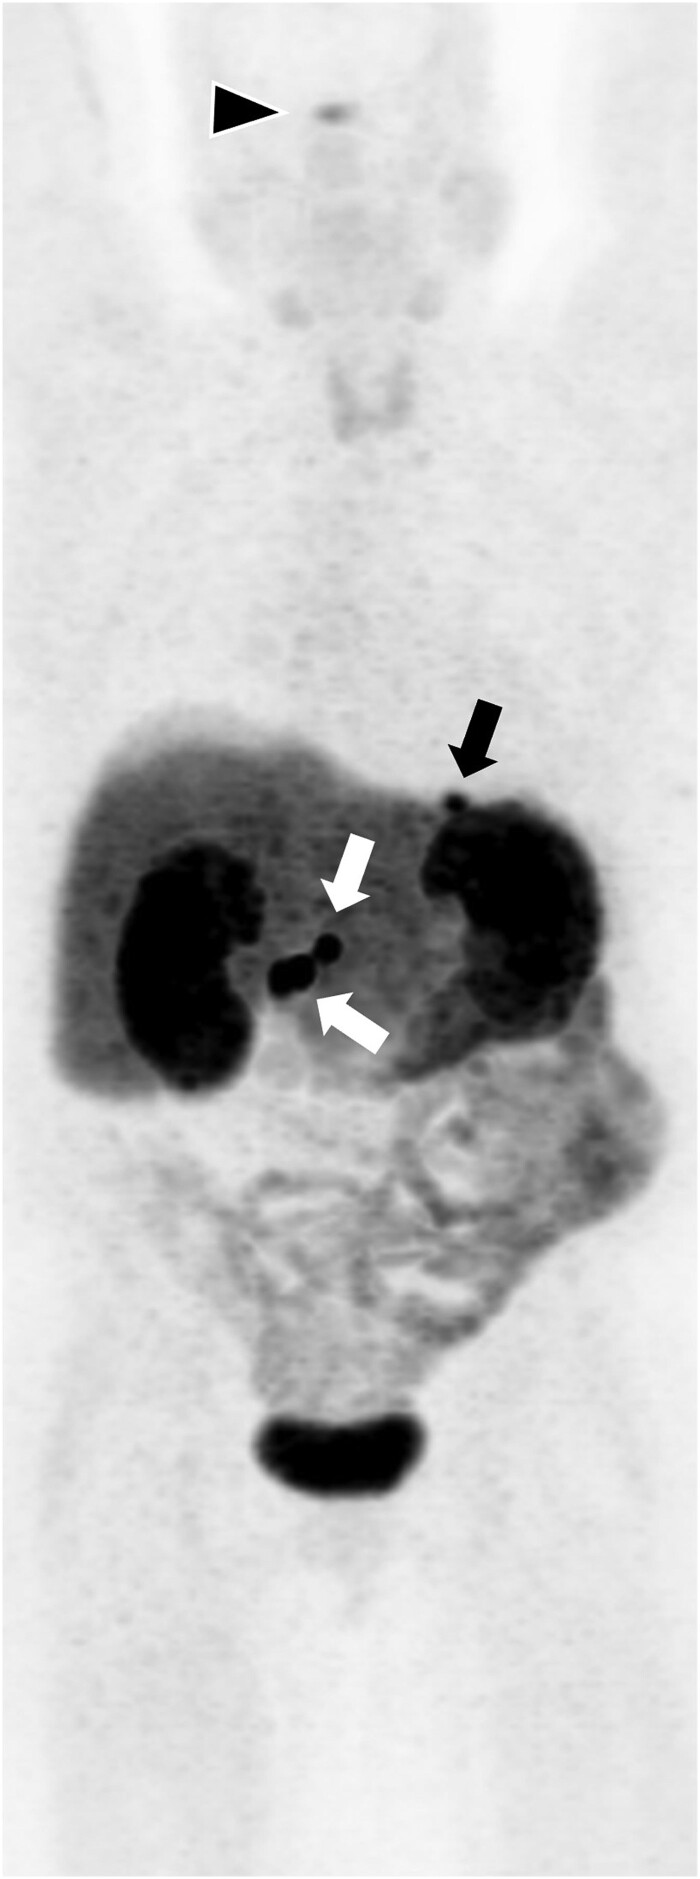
Maximal intensity projection image from the initial Ga-68 DOTA-TATE PET/CT obtained after pancreatic tumor resection demonstrates several somatostatin receptor–positive peripancreatic and porta hepatis lymph nodes (SUV max up to 27.1), measuring up to 1.3 cm in maximal dimension (white arrows). Increased tracer uptake is also seen in a small hepatogastric ligament lymph node (black arrow). Physiologic tracer uptake is seen in the pituitary gland (black arrowhead).
